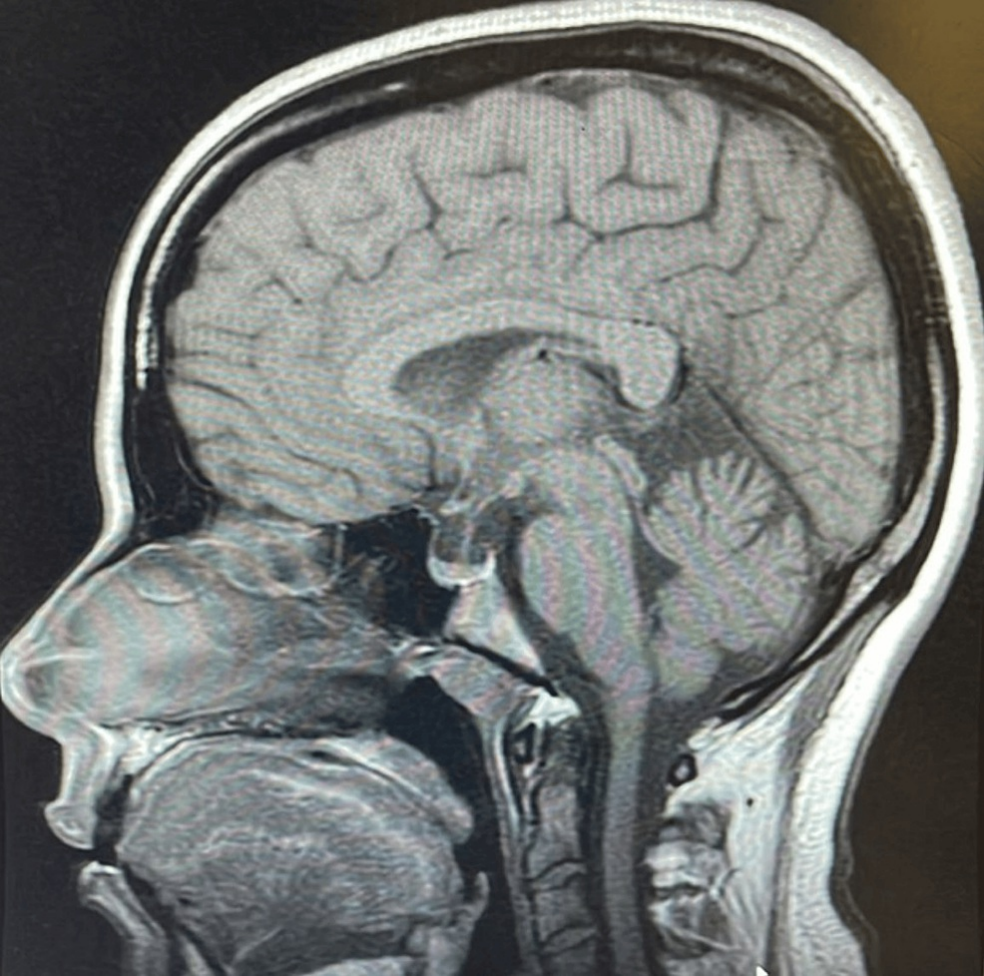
Sagittal T1 weighted MRI showing a partially empty sella
